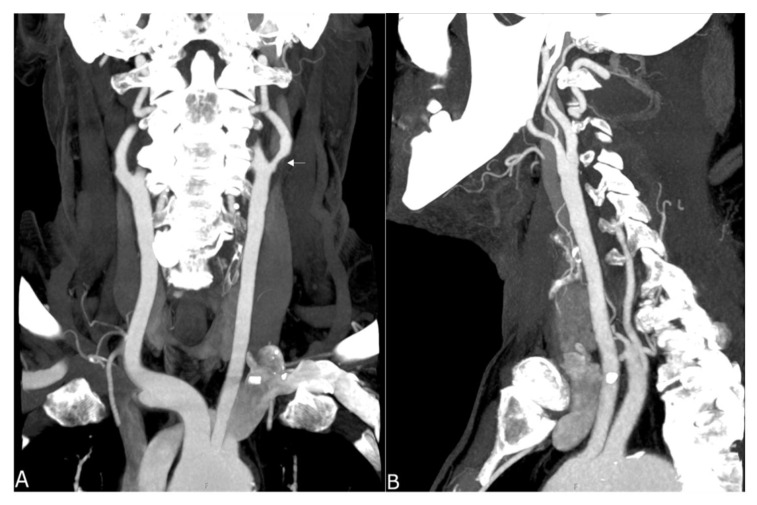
Maximum intensity projection of a supra-aortic UHR-CTA, (A) coronal projection showing an internal carotid artery plaque (white arrow) as well as contrast and delineation of, e.g., the thyrocervical trunk; (B) sagittal projection.
